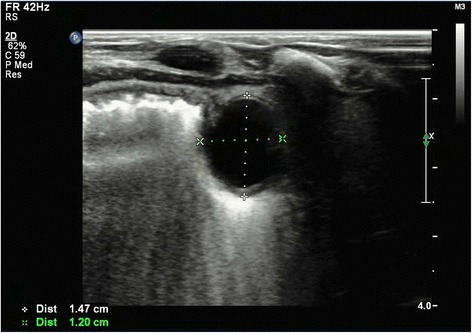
Ultrasonographic image of the gastric duplication cyst. It was 1.5 × 1.4cm and intimately attached at the greater curvature of the stomach
